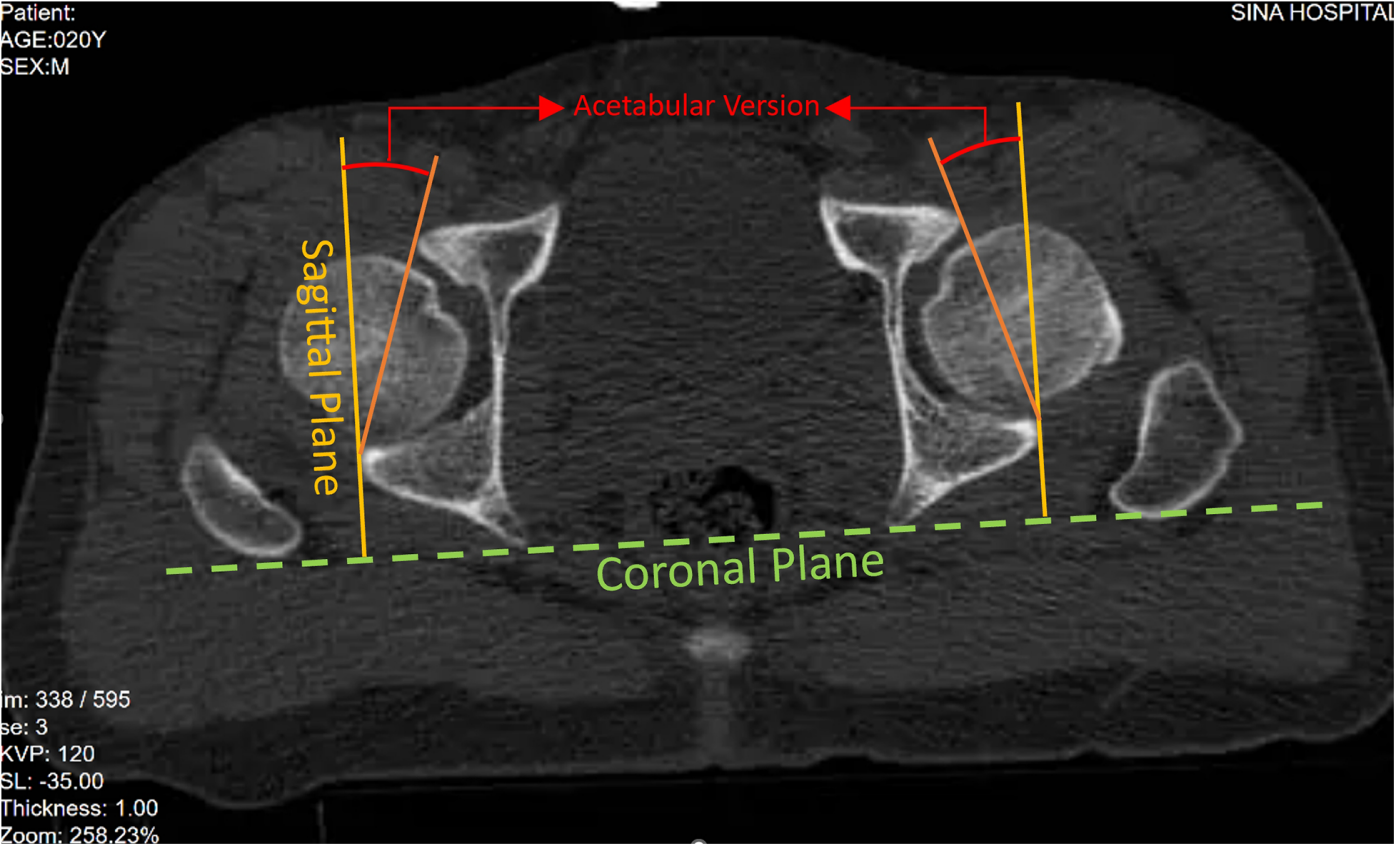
Measurement of acetabular version in equatorial plane of acetabulum CT-Scan.
